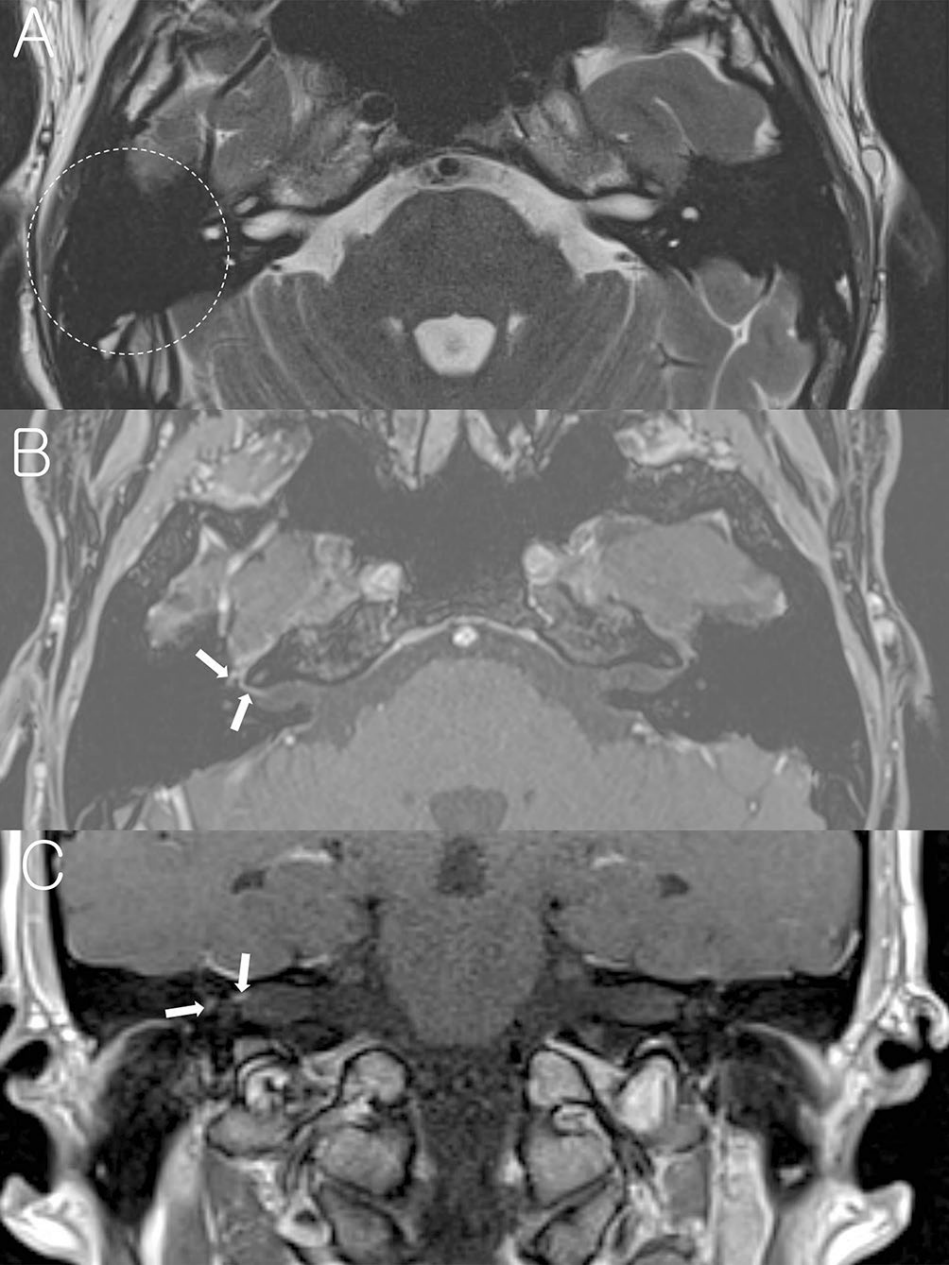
A representative case of the right Bell’s palsy with normal mastoid cavity in temporal bone MRI. (A) Non-contrast axial T2-weighted image shows normal mastoid cavity on the right side (dotted circle). (B) Post-contrast axial T1-weighted image at the Bill’s bar shows enhancement of the distal canalicular, labyrinthine, genu and proximal tympanic segment of the right facial nerve (arrows). (C) Post-contrast coronal T1-weighted image at the level of IAC demonstrates enhancement of the right facial nerve.
